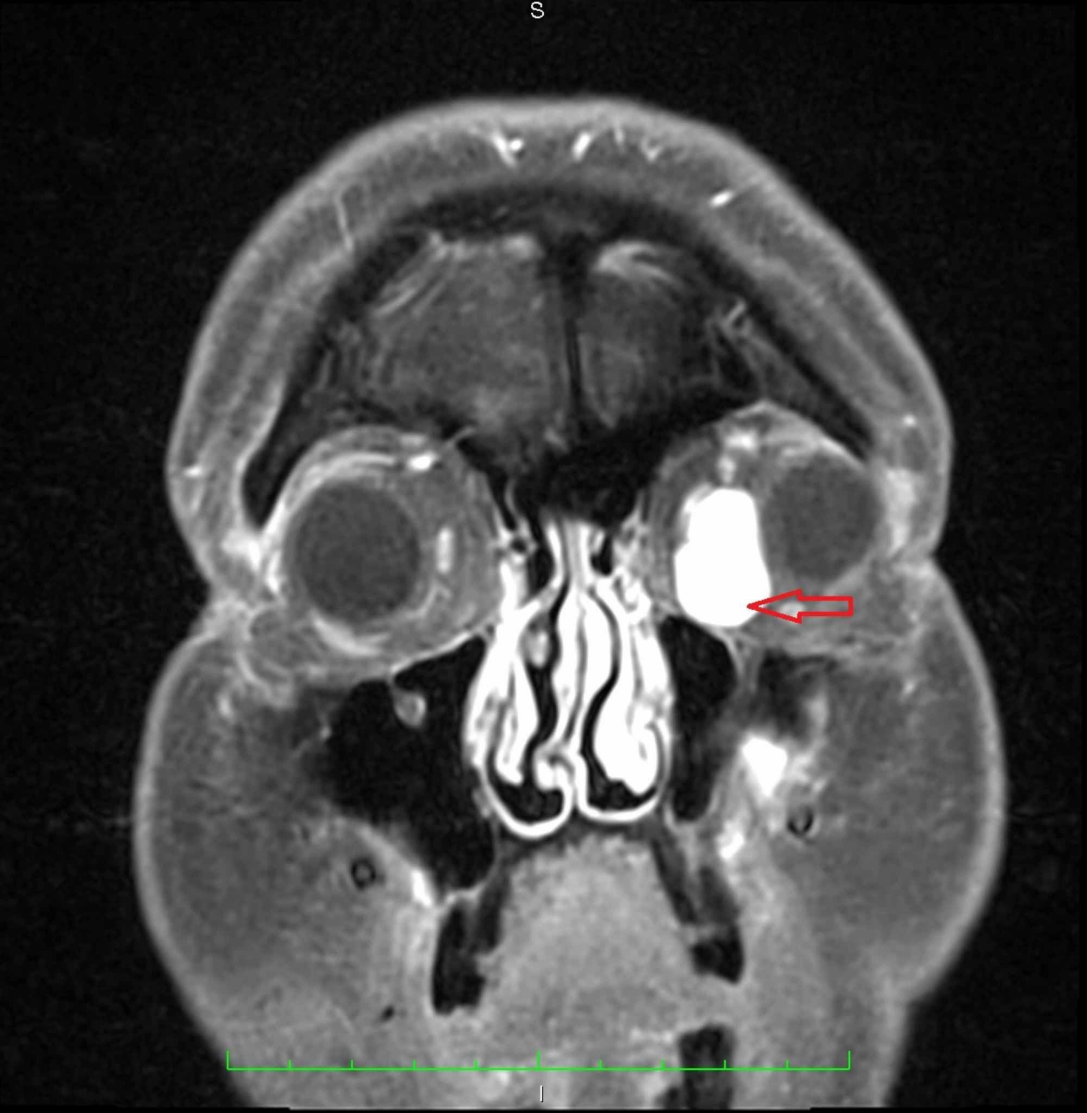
Coronal MRI of the orbits with contrast gives another view of the enhancing lesion within the left orbit
